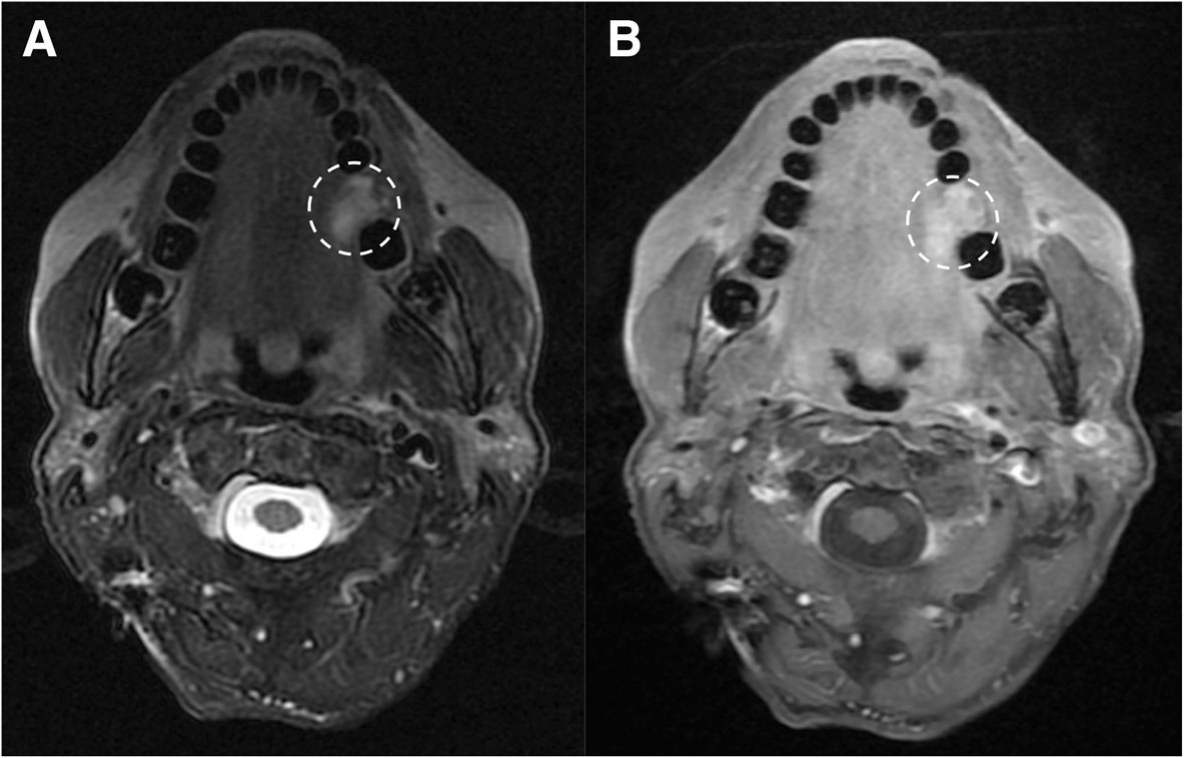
Neurofibroma of the lingual nerve. Patient with NF1. MRI T2-weighted fat-suppressed (a) and T1-weighted post-gadolinium (b) axial images, display an hyperintense and enhanced round mass along the course of the left lingual nerve (dotted circles)
